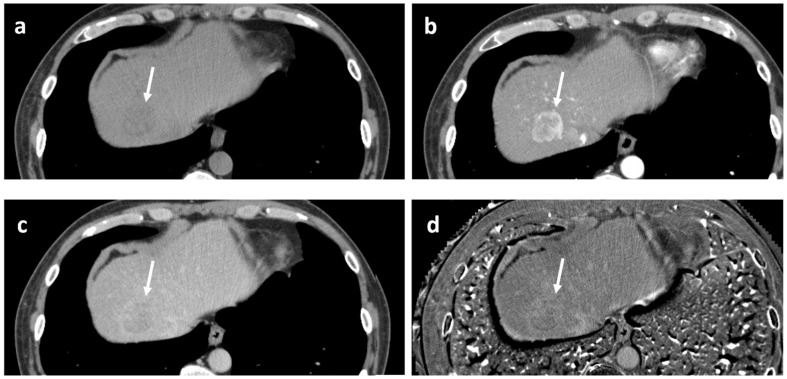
Example of positive washout case at the equilibrium phase. 53-year-old man with alcoholic liver disease. Pathologically, moderately differentiated hepatocellular carcinoma of confluent multinodular type, without complete fibrous capsule, was confirmed. Fatty change and lymphocytic infiltration were observed within the tumor. (a) Precontrast CT. The tumor exhibits low density (arrow). Measured density were 46 and 56 Hounsfield units for the tumor and the background liver, respectively. (b) Arterial phase CT. The tumor shows periphery-dominant enhancement (arrow). (c) Equilibrium phase CT. The tumor exhibits apparent “washout” (arrow). (d) Extracellular volume fraction (ECV) map reveals slightly higher value of the tumor, as compared to the background liver. Measured ECVs were 38.5% and 33.1% for the tumor and the background liver, respectively. In this case, although the ECV of the tumor is slightly higher than that of the BGL, it was considered that the lower precontrast density of the tumor, possibly due to fatty change, contributed to the positive EqP washout.
